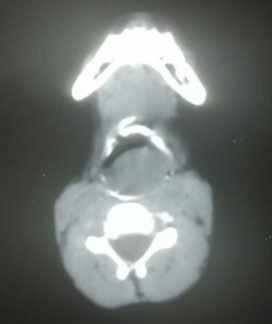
CT imaging mass during the first visit. showing a hyperdense inlet.
